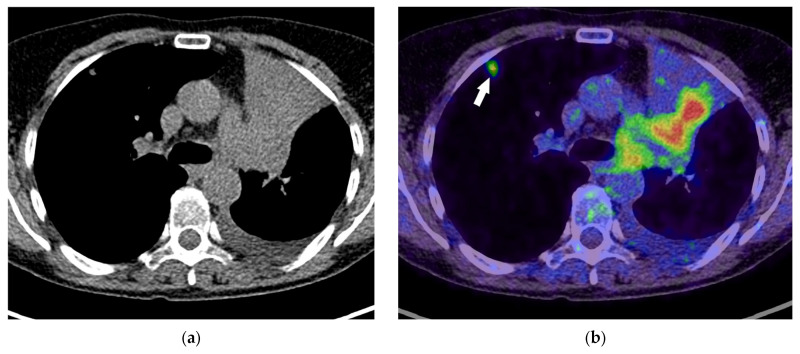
The role of PET/CT in differentiation between obstructive lung cancer and atelectasis: An axial non-contrast-enhanced chest CT image (mediastinal window) showing a left hilar mass accompanied by the atelectasis of the upper-left pulmonary lobe (a). A PET/CT image (b) confirming an FDG-avid lung cancer in the left hilum and allowing for better differentiation of the tumor from non-FDG-avid upper-left lobe atelectasis (b). There is an associated left-sided pleural effusion and an upper-right lobe metastasis (arrow), indicating M1a disease.
